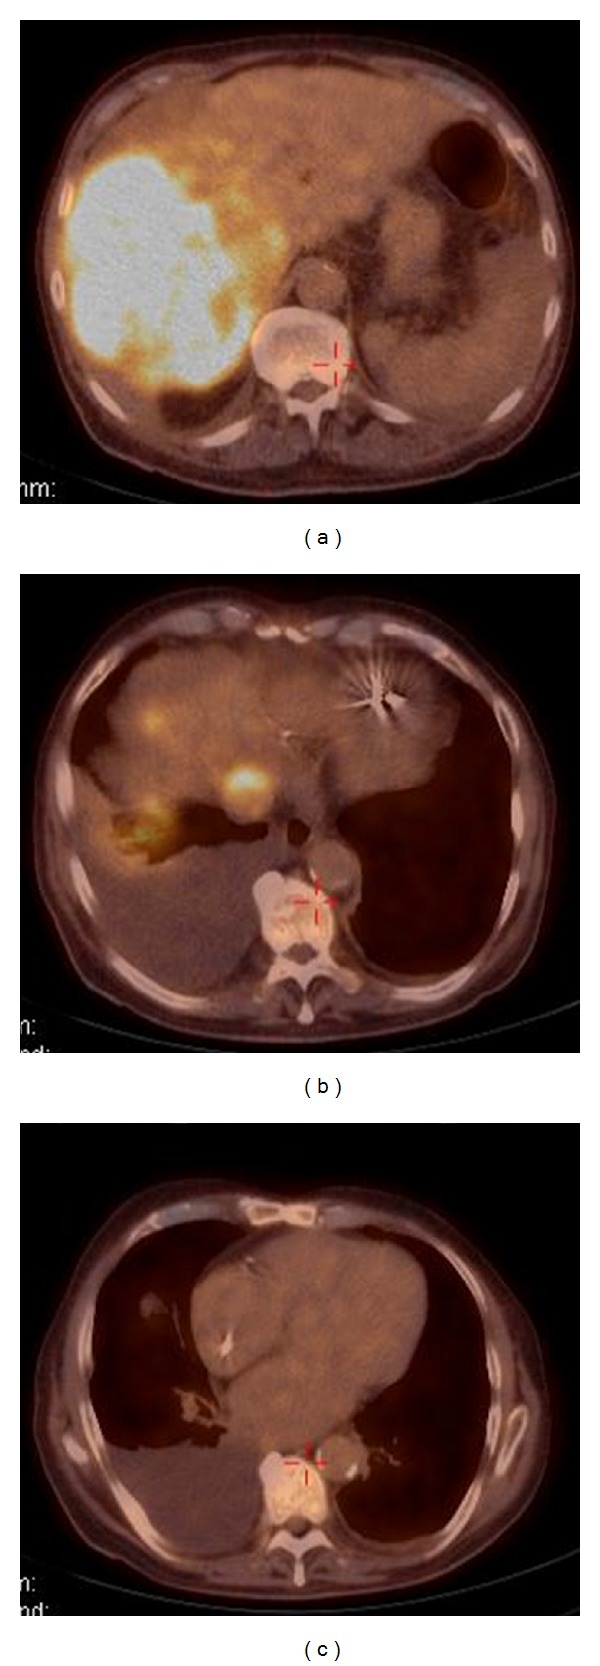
Positron emission tomography-CT.
